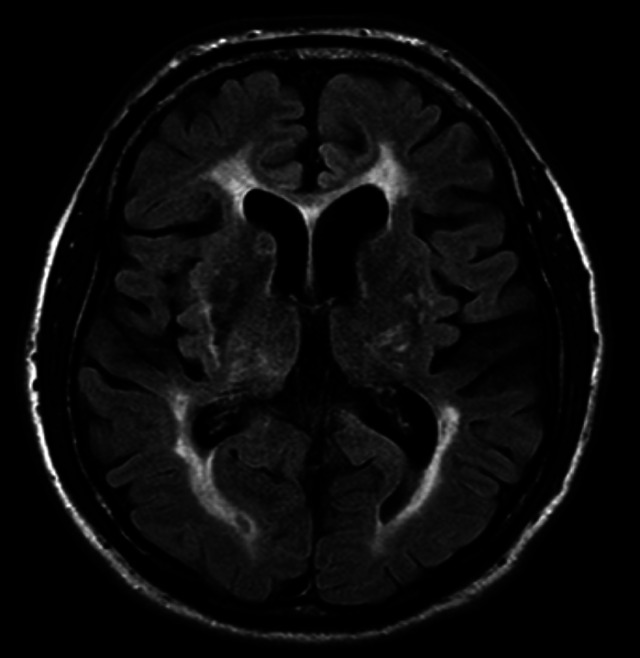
Vascular parkinsonism. Axial FLAIR MRI shows hyperintense foci involving the basal ganglia, thalamus, periventricular and subcortical white matter related to chronic small vessel ischemic disease.
